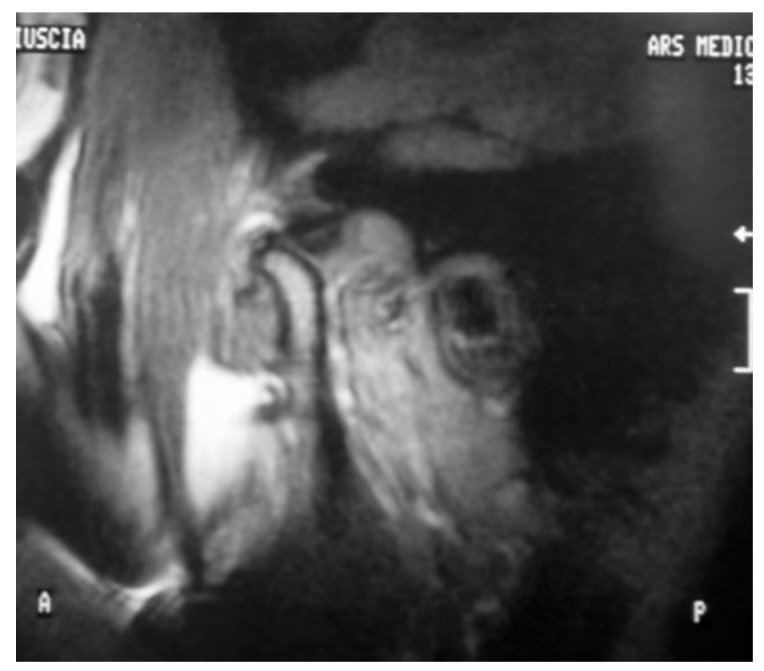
Magnetic Resonance Image after treatment T2. An improvement in disc position is observed.
